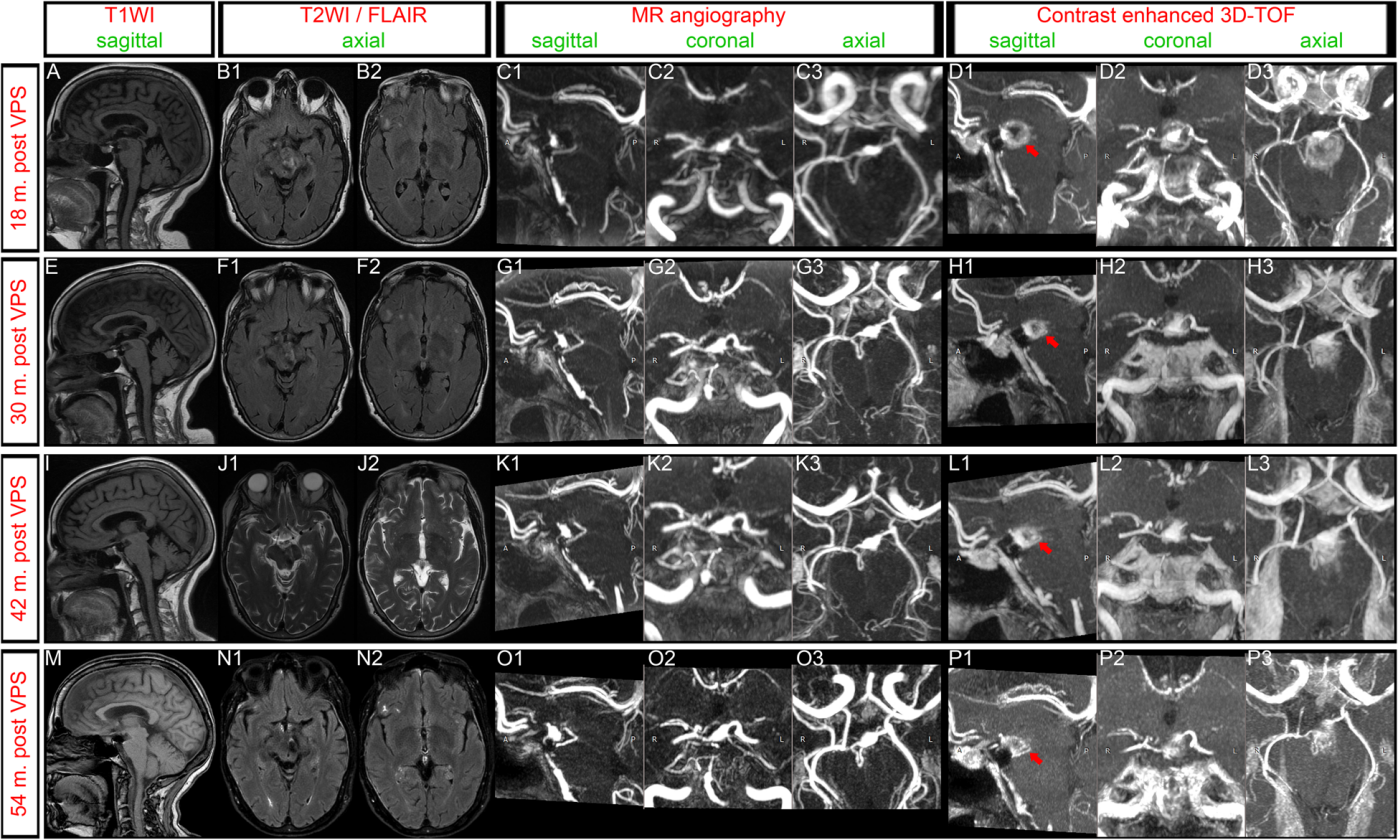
Follow-up magnetic resonance imaging (MRI) showed almost complete thrombosis of the lumen of the aneurysm and progressive reduction of the aneurysmal external contour. a, e, i, m: Sagittal T1-weighted images (T1WI) showed resolution of the intramural hematoma, no new intraluminal fresh thrombus and progressive decrease in the maximum external diameter of the aneurysm, thus alleviating brainstem compression. b, f, j, n: Axial fluid-attenuated inversion recovery (FLAIR) and/or T2-weighted images (T2WI) showed progressive resolution of brain edema. c, g, k, o: Sagittal, coronal and axial maximum intensity projection (MIP) reconstructions of magnetic resonance angiography (MRA) showed no filling of the aneurysm sac, and filling of the upper basilar apex via both posterior communicating arteries (PCoAs). d, h, l, p: Sagittal, coronal and axial MIP reconstructions of contrast enhanced 3-dimensional time of flight (3D-TOF). Although no filling of the aneurysm was found, a marked gadolinium enhancement is noted around the top of the basilar artery, representing the old intraluminal thrombus. The maximum external diameter of gadolinium enhancement decreased over the follow-up period (red arrows)
